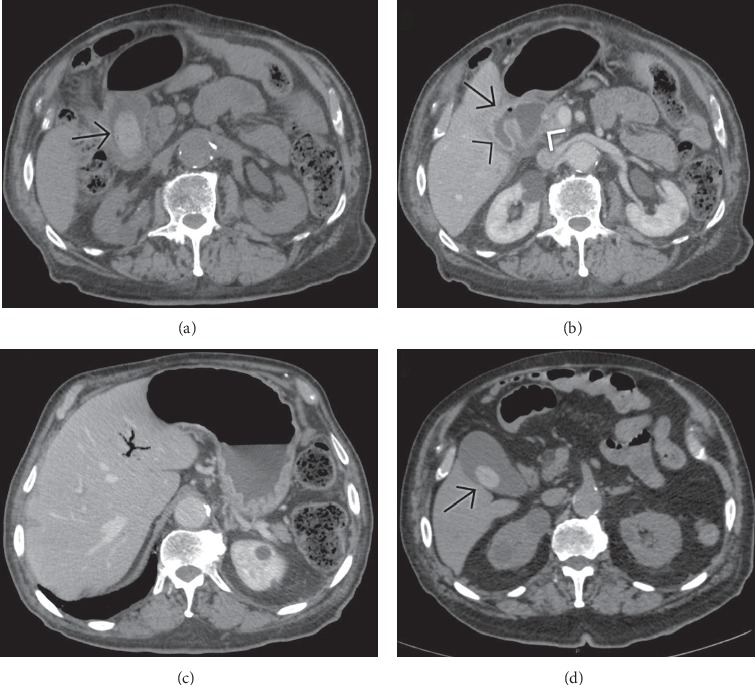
Unenhanced computed tomography in the same case of Bouveret's syndrome than Figure 4(a) shows a large slightly hyperattenuating filling defect (arrow) in the duodenal bulb. A fistula between the gallbladder (black arrowhead) and the duodenum (white arrowhead) is evident in (b) after intravenous contrast media administration. The association of ectopic gallstone visualization, pneumobilia (seen in (c)), and signs of intestinal obstruction (gastric overdistension visible in (c)) constitute Rigler's triad. In a previous unenhanced computed tomography (d), a smaller hyperattenuating calculus was detectable in the gallbladder: this finding represents a clue to the diagnosis of gallstone ileus.
